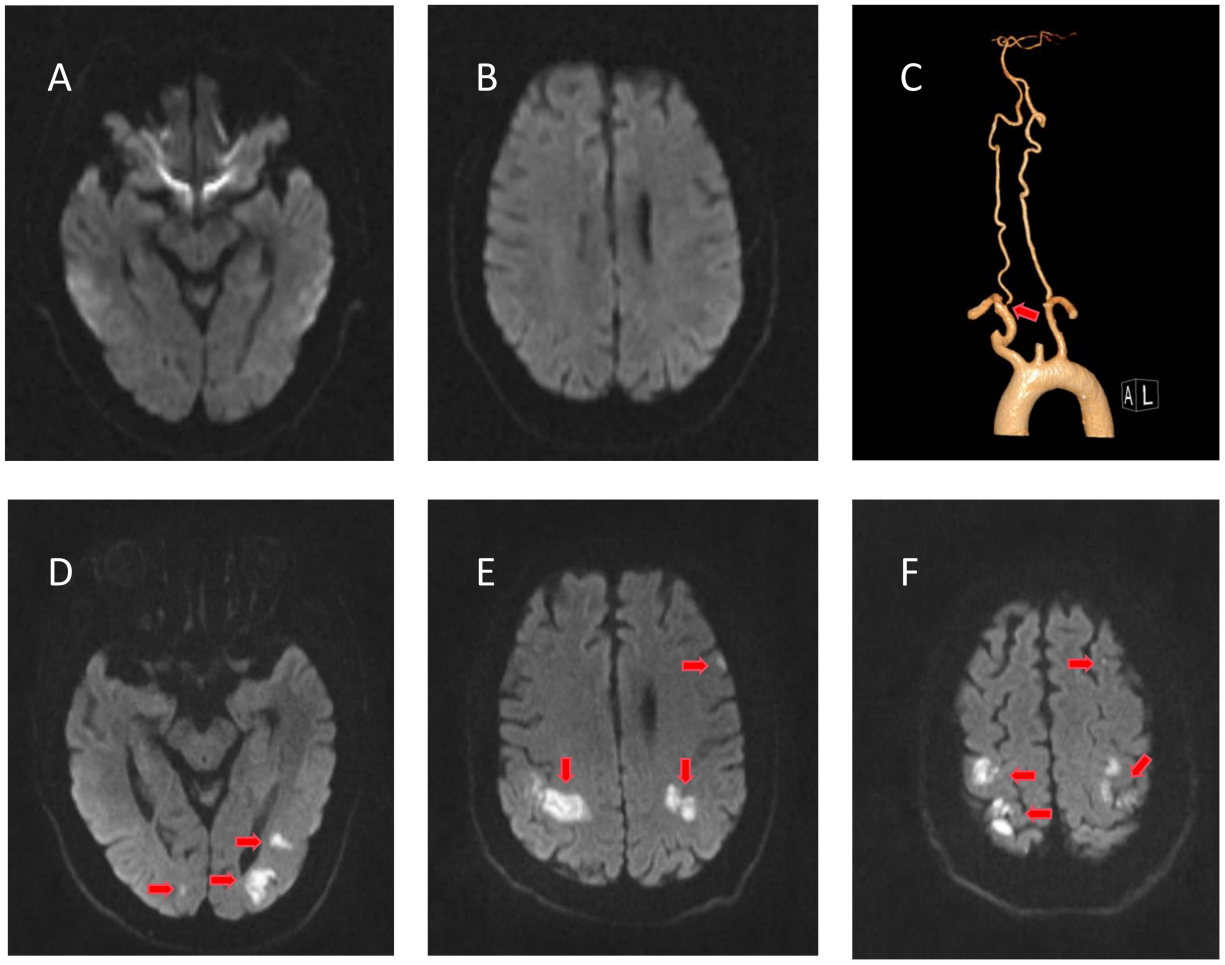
Radiological examinations of case 2 before and after intravenous immunoglobulin (IVIg). (A, B) No evidence of cerebral infarction before IVIg on diffusion-weighted imaging (DWI). (C) Computed tomography angiography showing right vertebral artery stenosis. (D–F) DWI of the bilateral parieto-occipital and left frontal lobes showing restricted diffusion of the lesion after IVIg.
